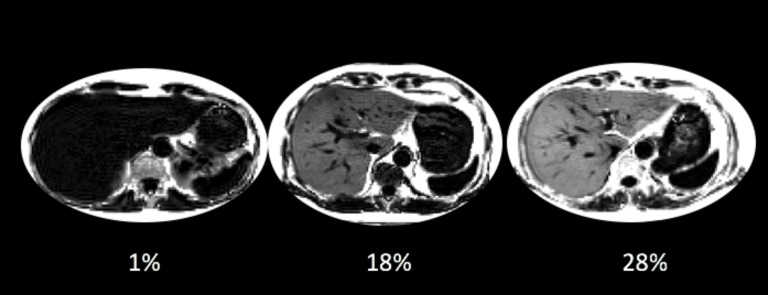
Representative MRI images from the UKBiobank cohort, showing individuals with 1%, 18% and 28% PDFF. Clear differences in the intensity of the liver can be seen, with the liver appearing brighter as PDFF increases.
